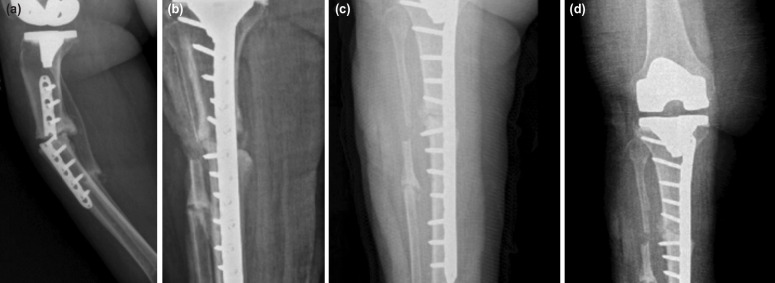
(a) Implant failure after operation for Felix type 3 periprosthetic fracture. (b) Internal fixation by means of iliacautogenous grafting and locking compression plate (c) 16th week radiography. (d) 24th week radiography.
